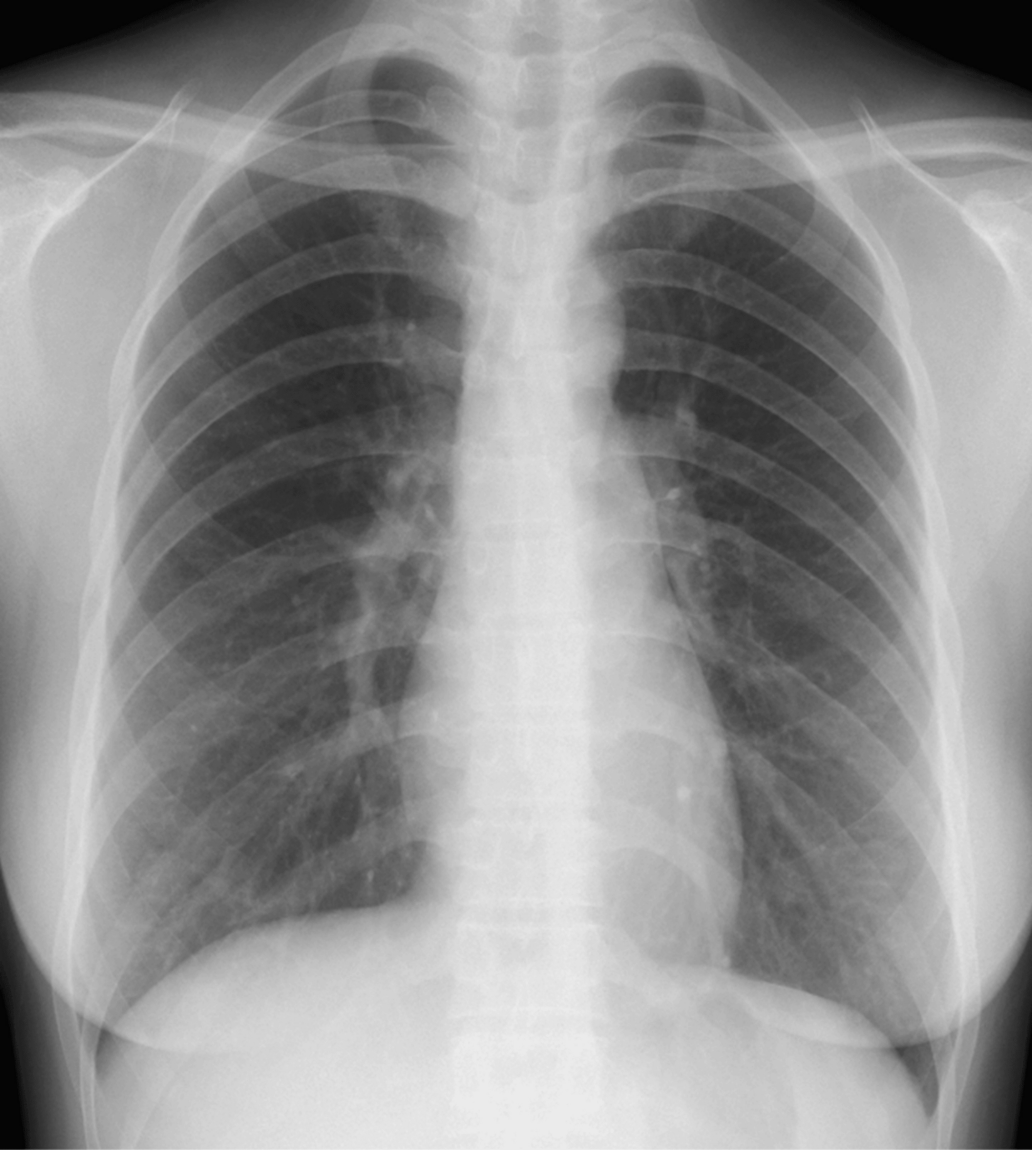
Chest radiograph of the second case No significant findings were evident.
